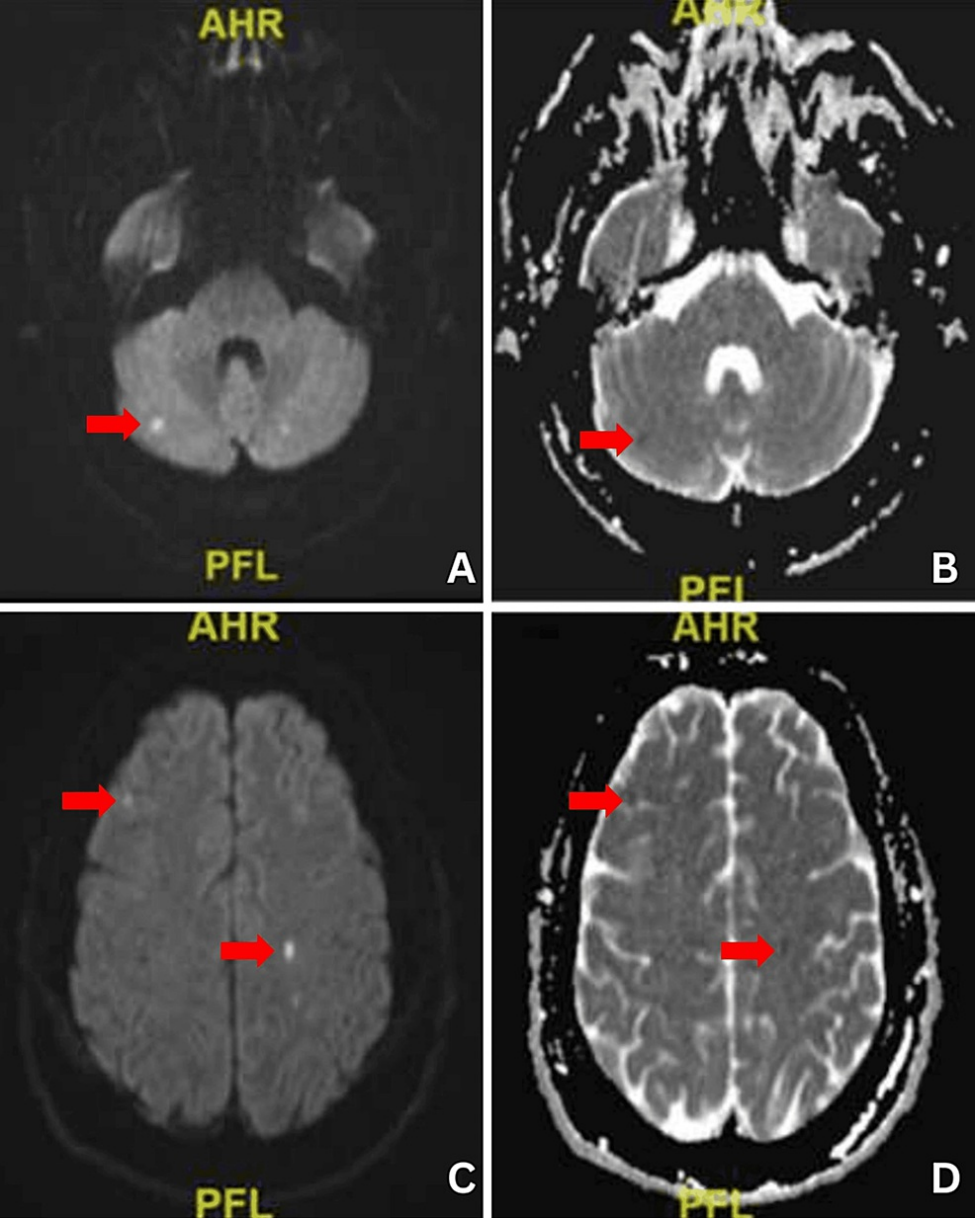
Diffusion-weighted (DWI) axial MRI with arrows demonstrating both supratentorial and infratentorial hyperintense foci that are correlated to hypointense foci in apparent diffusion coefficient (ADC) c/w multiple small acute infarctions
